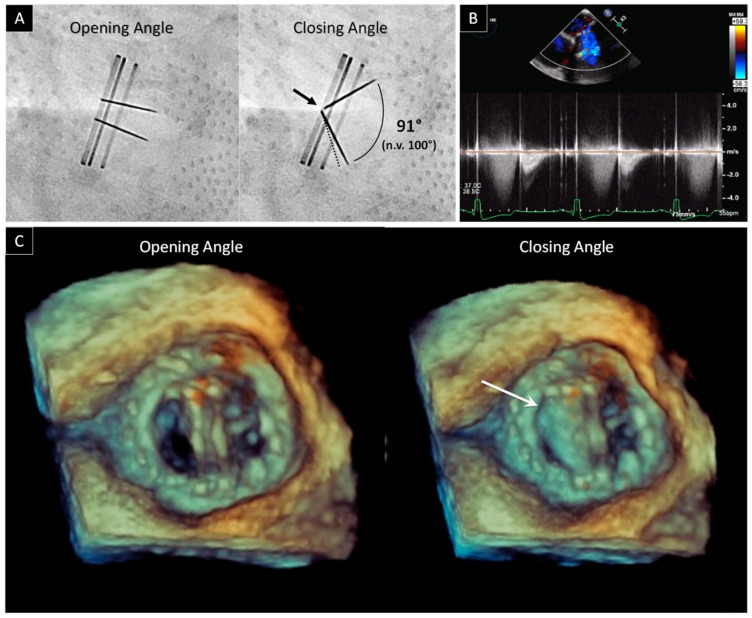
Bileaflet mechanical mitral valve obstruction. (A) Fluoroscopic evaluation of normal opening and abnormal closing angles in a patient with prosthetic mitral valve dysfunction. The dotted line refers to the normal leaflet closure. The abnormal leaflet closure was confirmed by the lack of leaflets contact at the hinge area (black arrow). (B) Holosystolic regurgitation at continuous wave Doppler by TEE. (C) 3D view of prosthetic mitral valve from atrial side. During systole, only one leaflet closes (white arrow).
